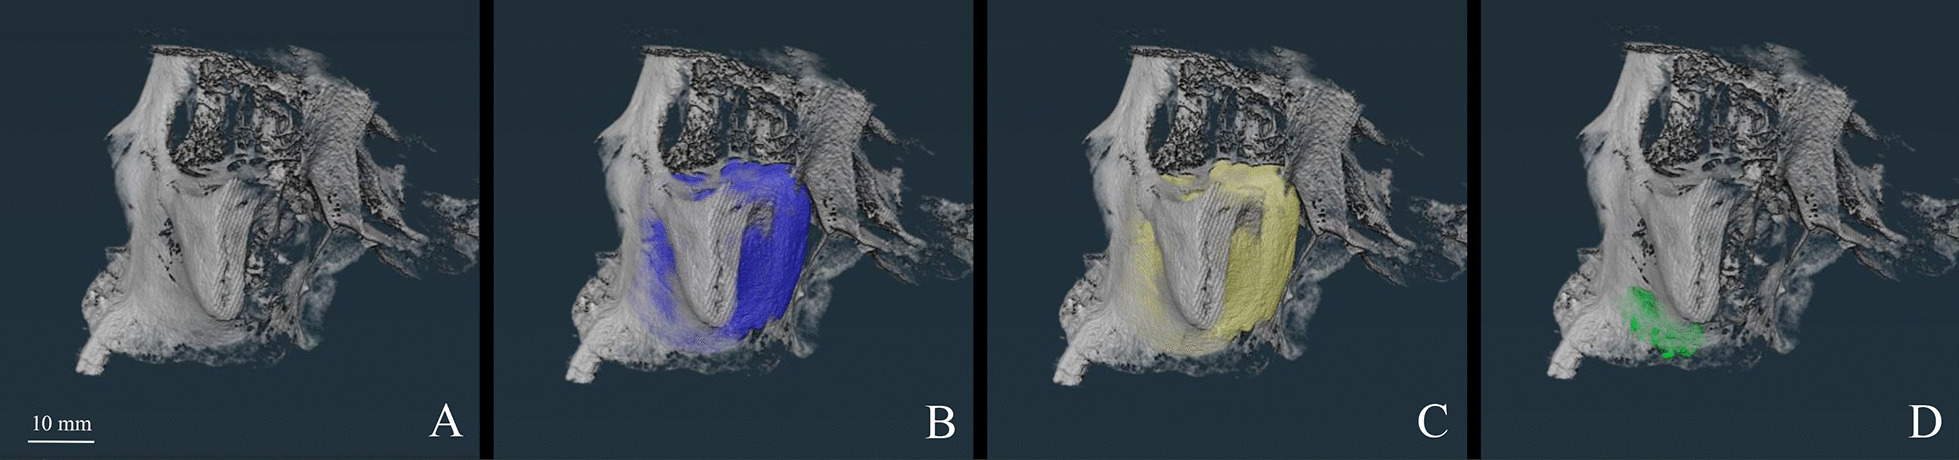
Three-dimensional display of the measurement. A Three-dimensional reconstruction of CBCT data; B The whole sinus cavity (purple); C The air cavity (yellow) of the preoperative sinus; D The grafts (Green) in the sinus cavity extracted from post-operative CBCT
